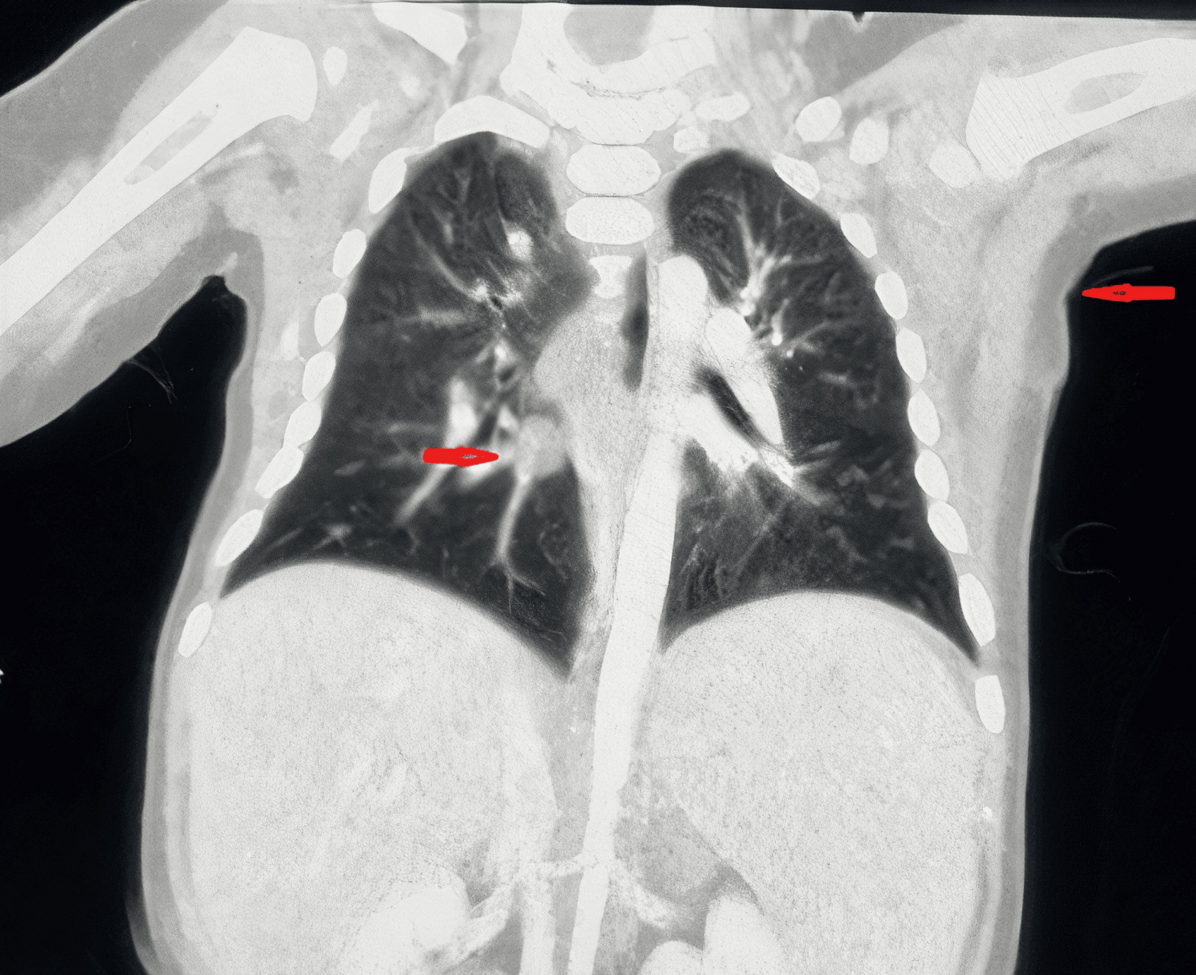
CT chest (sagittal view) showing left axillary ill-defined mass lesion with middle lobe small pulmonary nodule
